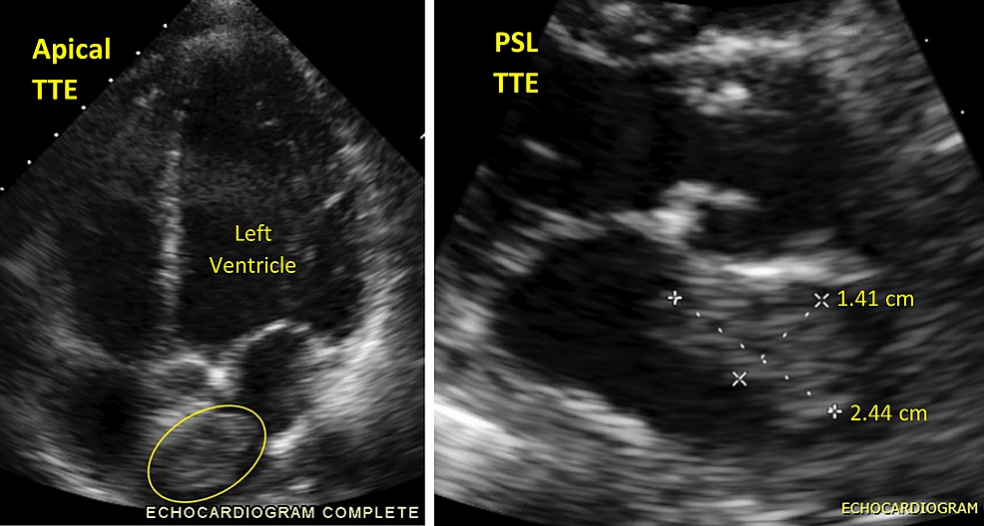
Transthoracic echo Left image: apical four-chamber view with the left atrial mass outlined. Right image: magnified parasternal long axis centering the aortic valve and left atrium with tumor measurements
